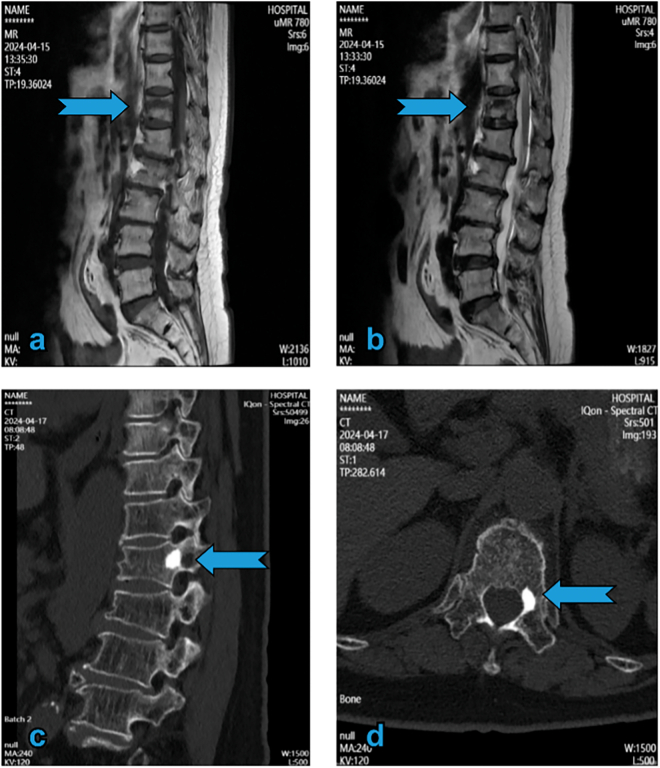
MRI of T1 and T2 sequences and preoperative CT. (a and b) Revealing vertebral compression fracture with hypointensity in T1 and hyperintensity in T2 (arrow). Preoperative CT (c) showing sclerosis lesions in the inner wall of the left pedicle in T12 (arrow). MRI: magnetic resonance imaging; CT: computed tomography.
